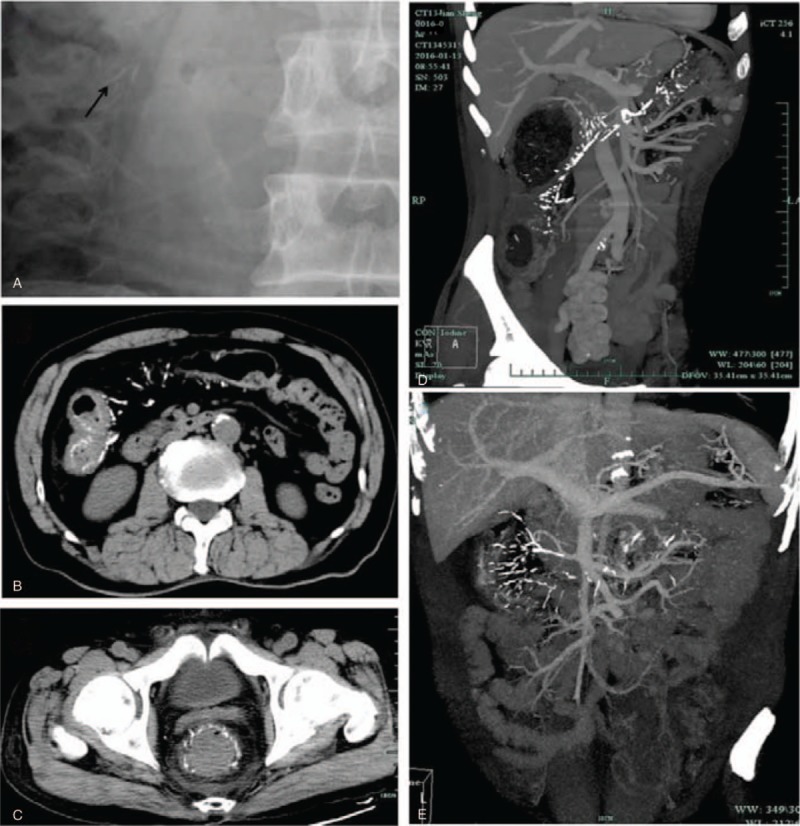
Characteristic radiologic findings. (A) Plain abdominal X-ray showed thread-like calcifications in the right hemicolon region (arrowheads). (B) On abdominal computed tomography (CT), wall-thickening and calcifications within the bowel wall and adjacent mesentery were observed. (C) A very rare phlebosclerotic colitis patient, linear calcifications extended into rectum. (D, E) Three-dimensional reconstruction of CT angiography depicted the precise extent of calcification.
